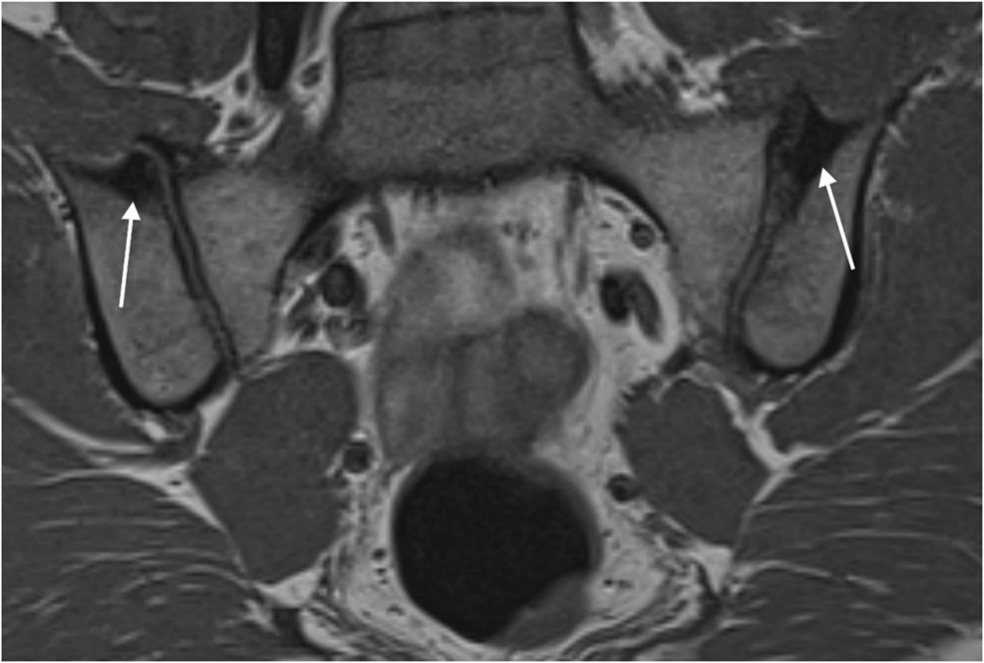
Oblique coronal T1-weighted MRI of SIJ of a 47-year-old woman with mechanical low back pain (no SpA) and childbirth history: two children, one of which delivered naturally, the other by C-section. The last delivery was 22 years and 8 months ago. Image depicts substantial subchondral sclerosis (arrows) in the upper quadrant of both SIJ in the os ilium, representing a sclerosis score of 2 on the right side and 3 on the left side, respectively. *C-section* cesarean surgery, *SIJ* sacroiliac joint, *SpA* spondyloarthritis
